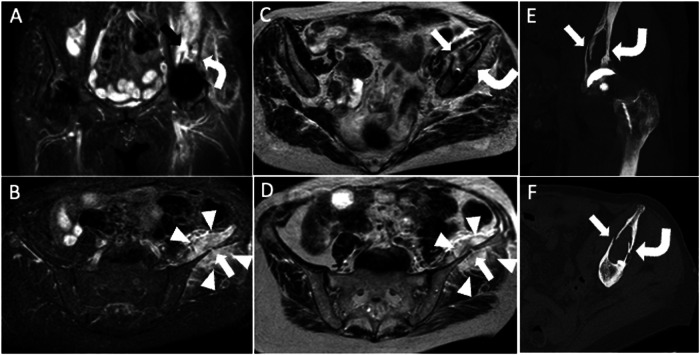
77-year-old female with hip arthroplasty, periprosthetic osteolysis, and fracture. Coronal STIR (A), axial STIR (B), and axial T2-weighted (C, D) images show acetabular periprosthetic osteolysis (curved arrows) and fractures that reach the iliac wing (arrows). Also, note the hematoma (arrowheads) within the gluteus minimus and iliac muscles. Coronal (E) and axial (F) CT images well depict periprosthetic osteolysis (curved arrows) and cortical disruption (arrows)
